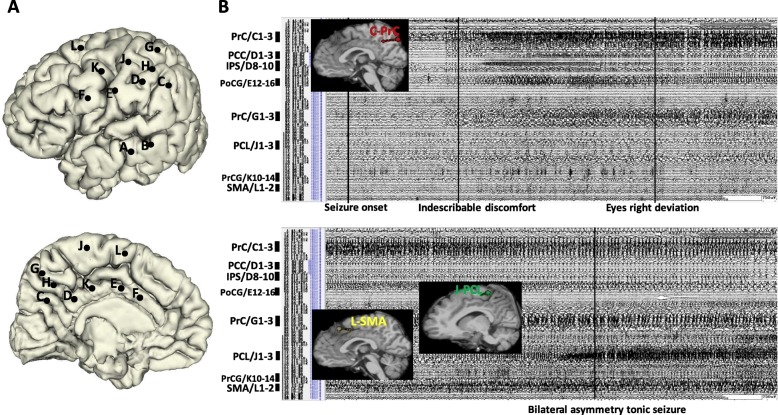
a SEEG electrode implantation on lateral and medial view of three-dimensional (3D) brain of Patient 2. b Ictal SEEG trace and two-dimension (2D) T1-weighted sagittal images with 3D electrodes showing the key target points. It shows that the seizure onset was recorded from precuneus (PrC) (medial contacts of electrode C1–3) with no clinical sign being observed. When the ictal activity spread to PCL (medial contacts of electrode J1–3), PrCG (lateral contacts of electrode K10–14), and SMA (medial contacts of electrode L1–2), the clinical sign of bilateral asymmetry tonic seizure appeared
